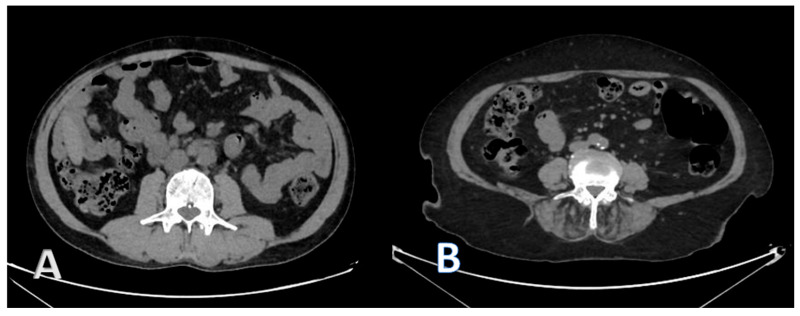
CT image at the L3 level of a recipient with normal muscle mass and quality (A) and a recipient with sarcopenia and myosteatosis (B).
